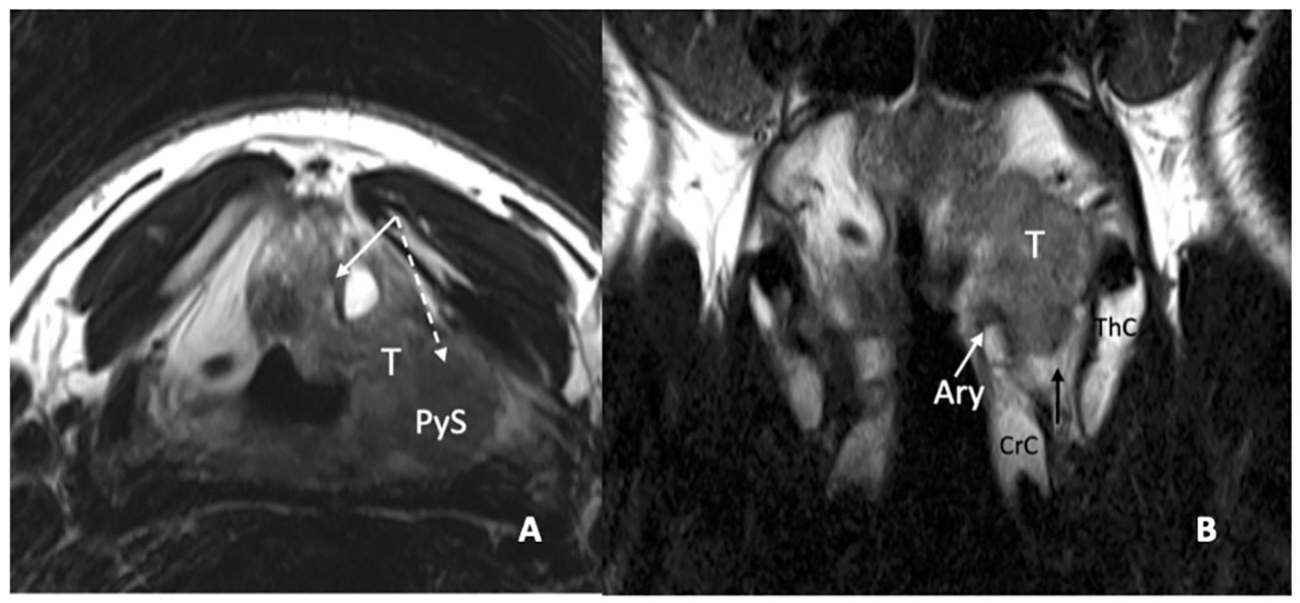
MRI T2-weighted images. Axial plane (A): at supraglottic level, the lesion (T) involves the pre-epiglottic space (white arrow) and the superior paraglottic space (PGS) on the left (dashed white arrow). The ipsilateral piriform sinus (PyS) is invaded. Coronal plane (B), the left transglottic tumour (T) mainly develops in the supraglottis, surrounds the ipsilateral arytenoid (Ary), partially sclerotic, and spreads towards the lower-posterior portion of the PGS, between the arytenoid and the thyroid cartilage (ThC). The inferior PGS (black arrow) is enlarged and shows high signal due to peritumoral inflammatory changes.
